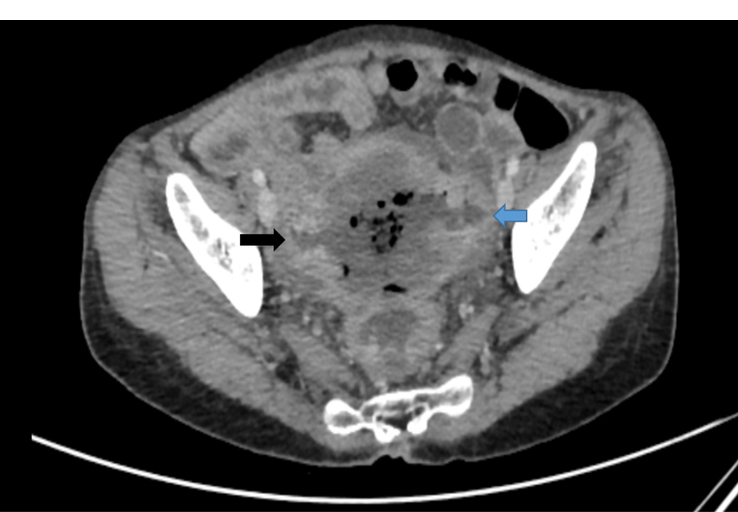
Axial section of contrast-enhanced computed tomography (CECT) showing two recto-jejunal fistulas, on the right (black arrow) and left (blue arrow) side.
